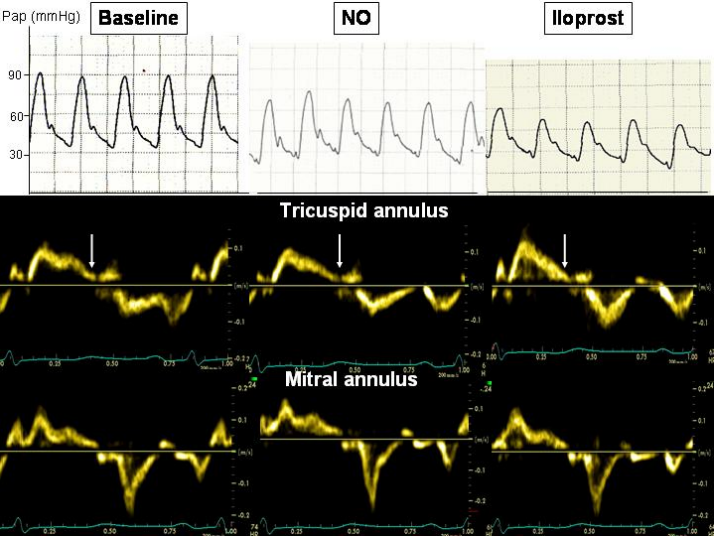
Pulmonary artery pressure (top) and pulsed-tissue Doppler traces at the tricuspid annulus (middle) and at the mitral annulus (at the bottom) before and during the inhalation of iloprost. The white arrows show the pulmonary valve closure.
